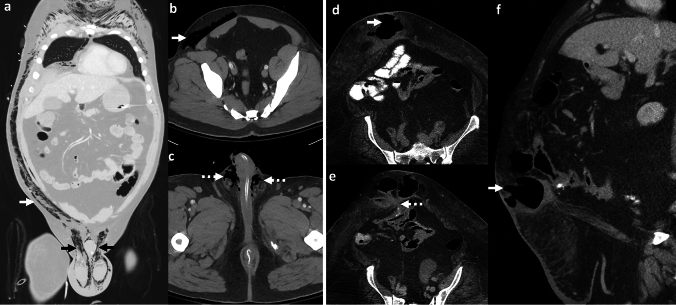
Pitfalls for Necrotizing fasciitis of abdominal wall and Fournier's gangrene. a–c Post-trauma or surgery: A 24-year-old with trauma and right flail chest. CT images show extensive subcutaneous emphysema (solid white arrow, a,b) with air tracking down to the scrotum outlining bilateral inguinal scrotal regions (black arrows, a; dotted white arrows, c). Unlike necrotizing fasciitis, air respects fascial planes, and there are no associated inflammatory changes. d–f Enterocutaneous fistula: A 57-year-old with swelling in the anterior abdominal wall. CT shows air fluid levels in the anterior abdominal wall with surrounding fat stranding (solid white arrows, d, f) and underlying enterocutaneous fistula (dotted white arrow, e)
